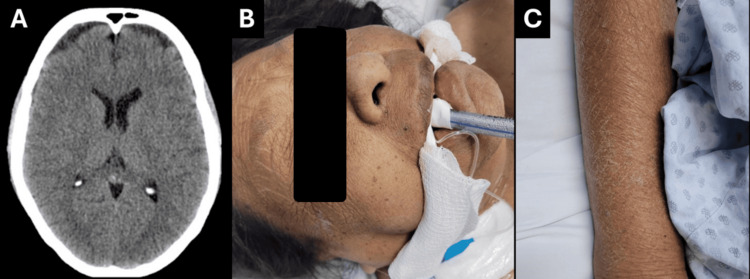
Clinical presentation of myxedema coma at admission. (A) Non-contrast brain CT showing no structural abnormalities. (B) The patient on arrival to the intensive care unit, showing characteristic generalized facial puffiness, macroglossia, dry coarse skin, and myxedematous features while under mechanical ventilation for hypoventilation and altered mental status. (C) Xerotic, thickened skin with scaling and rough texture on the forearm, consistent with severe hypothyroidism.
